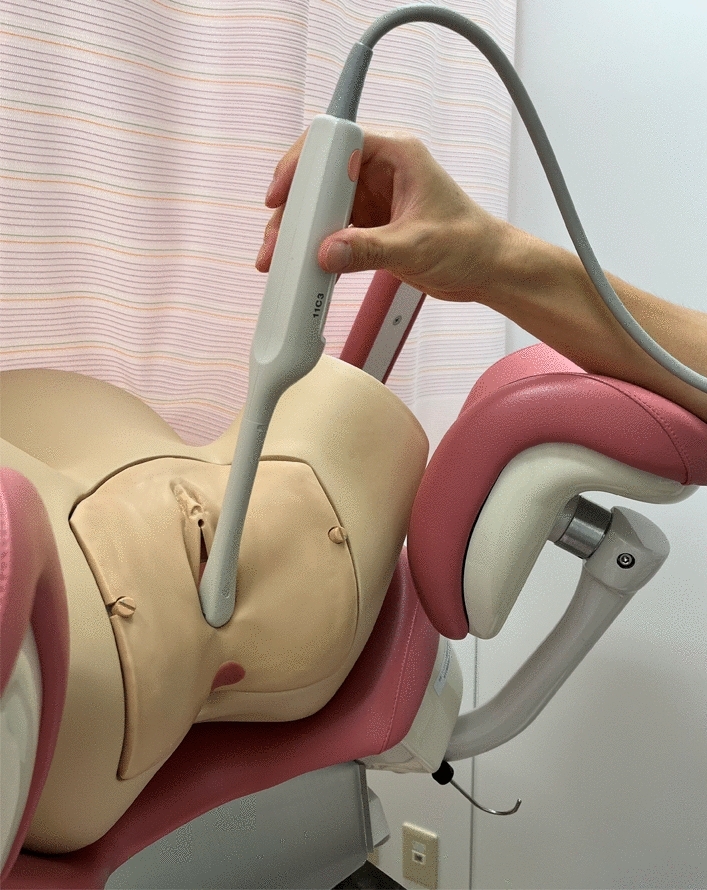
A transvaginal ultrasound probe is placed on the posterior vaginal wall
